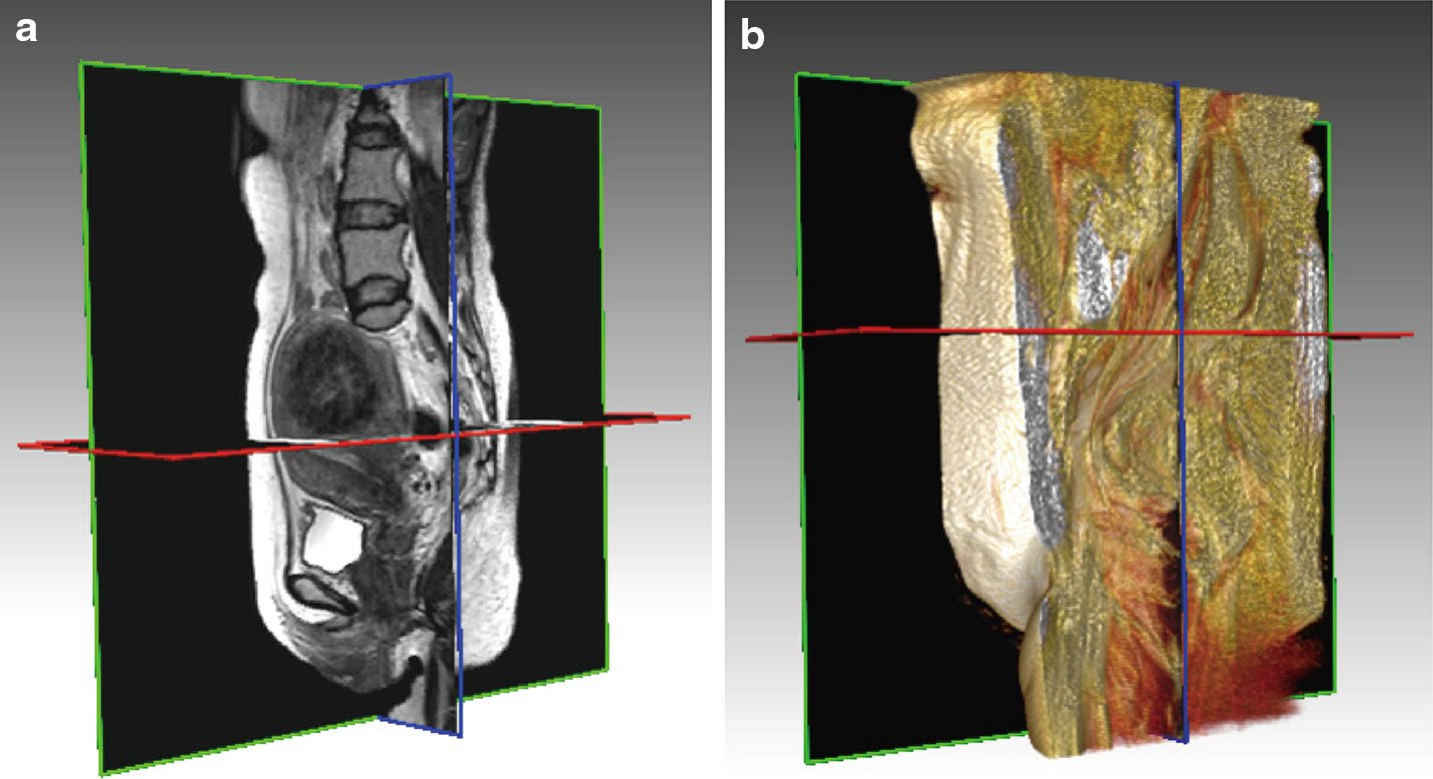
Advanced visualization by combining 2D slice and 3D rendering views. a 2D cross-sectional views of uterine MR images provide the advantage of viewing 2D images in different planes. b A combination of 2D and 3D views to provide visualization of the uterine structures from different directions and with scaling. The positional relationship of the uterine structures with respect to others organ nearby and the types of fibroids can be understood easily with the combination.
